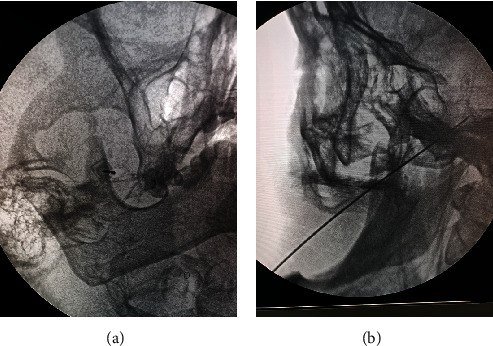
The surgical process of pulsed radiofrequency. (a) Adjust the appropriate angle of the fallopian tube to expose the foramen ovale, and puncture it into the foramen ovale with a radiofrequency needle under X-ray guidance. (b) Display the depth reached by the radiofrequency needle tip under lateral X-ray fluoroscopy.
